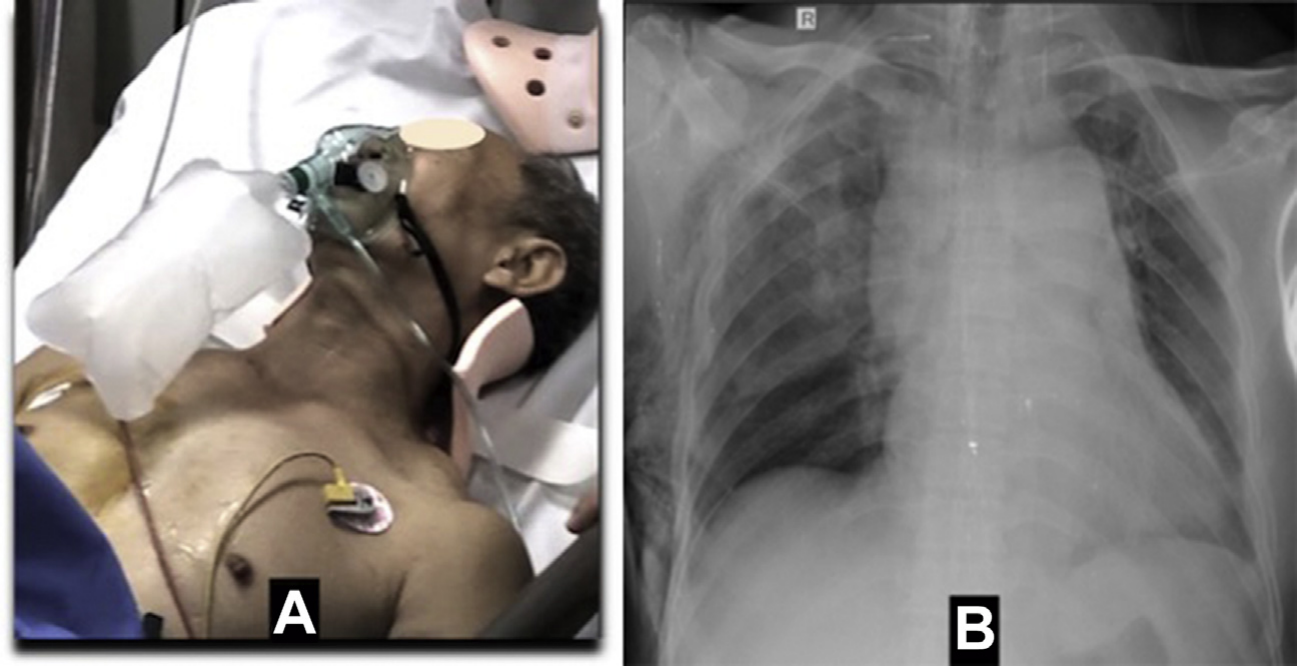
Initial Findings (A) Patient with unremarkable thoracic injury. (B) Chest radiograph revealing large mediastinum, right subcutaneous emphysema, rib fractures, and pneumothorax.
